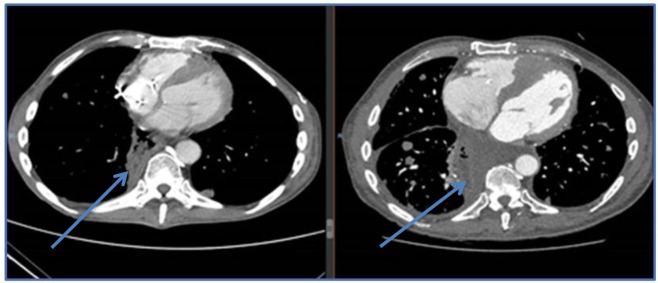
CT images of esophageal sarcoma metastatic with cardiac infiltration and cardiac failure, right image is on exogenous L-T4, left image is post L-T4 discontinuation and oral cyclophosphamide. Patient improved clinically and was discharged from intensive care.
